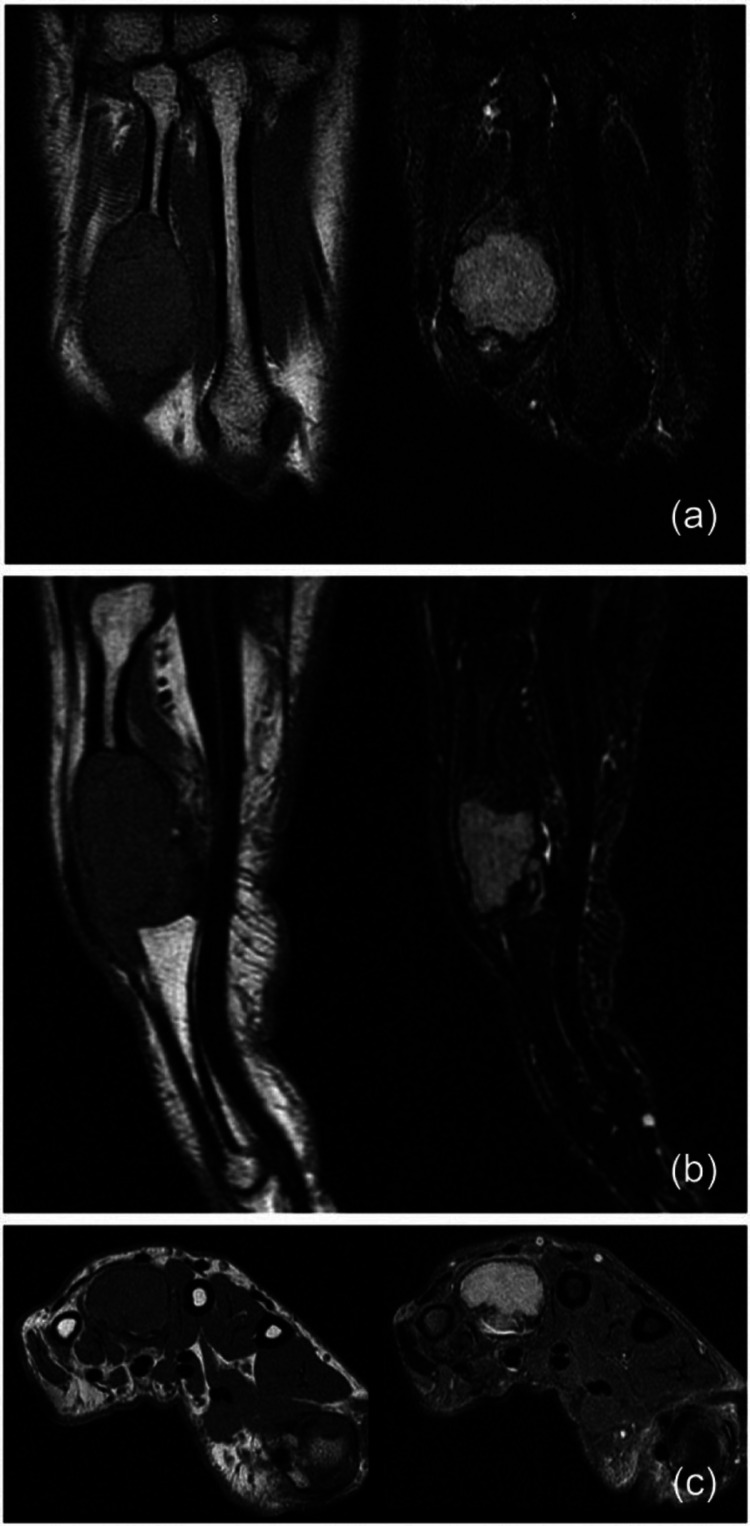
MRI cuts showing multiple lytic lesions along with a pathological fracture of the left 4th metacarpal.
